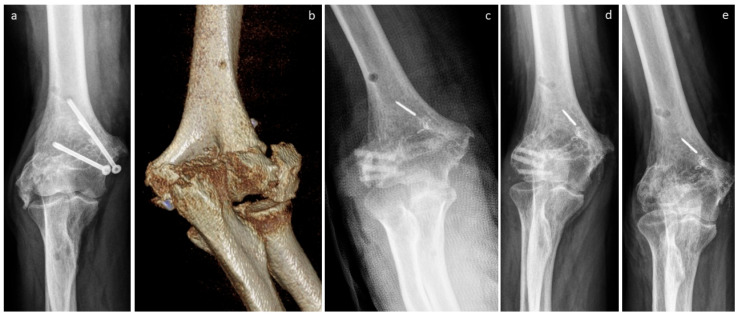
The non-union of the epicondyle of the lateral humerus of a patient. (a): Presurgical X-ray; (b): pre-surgical CT; (c): non-union was treated with three Shark Screws^®^. Union was observed after 10 weeks. (d): Four months after surgery. (e): A total of 21 months after revision, the Shark Screws^®^ are totally remodelled into the host bone.
